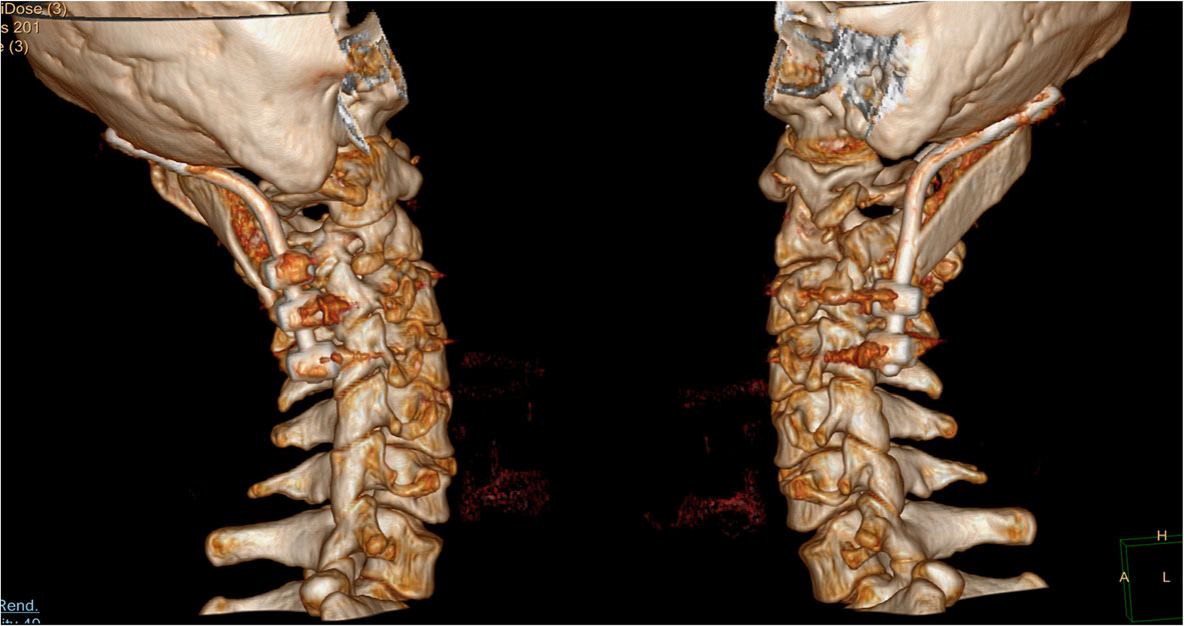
Lateral 3D-CT examination 5 years after operation showed that the internal fixation position was good, and the physiological curvature of the cervical vertebra was maintained well
